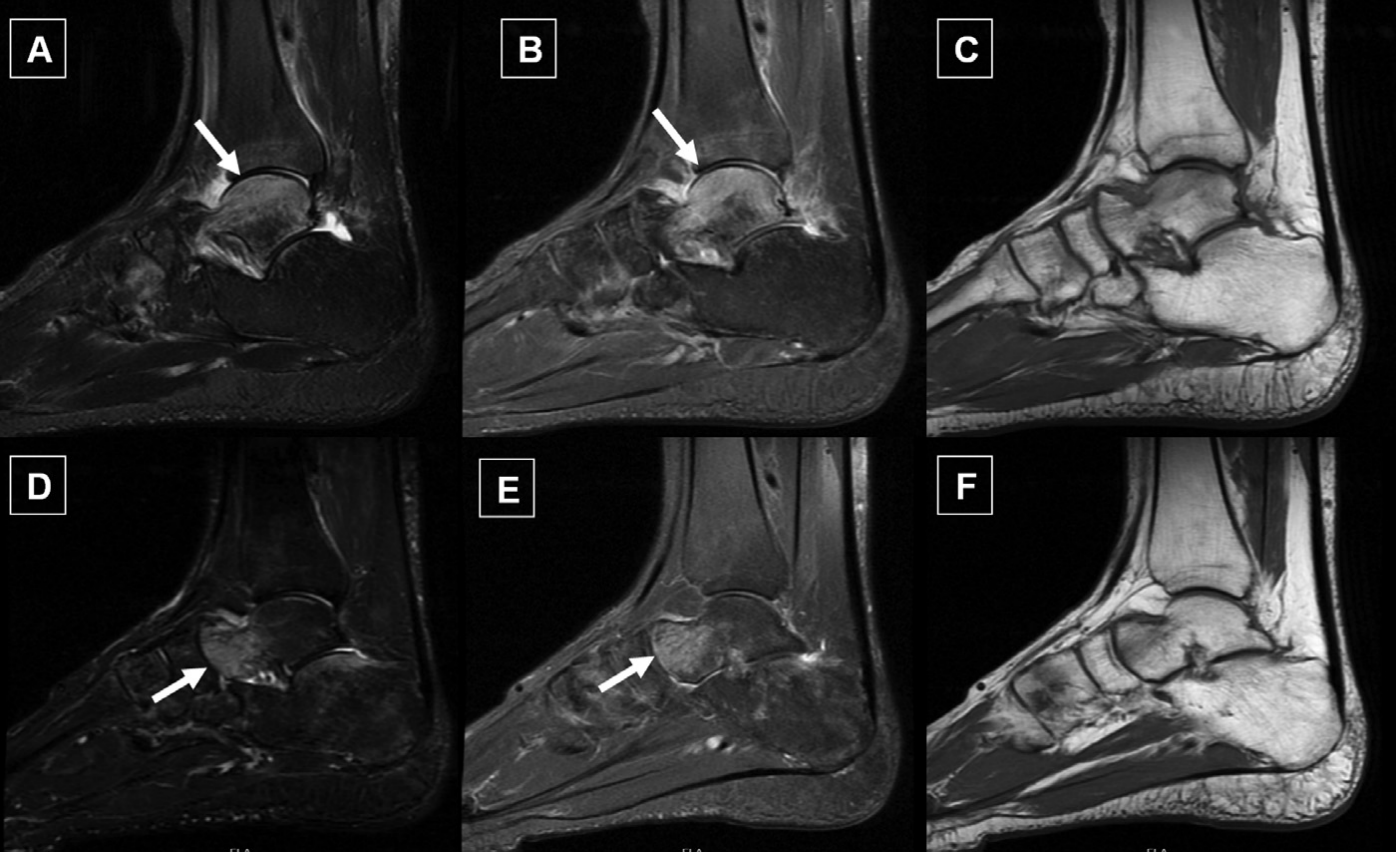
Sagittal MRI planes: (A) STIR, (B) PD, and (C) *T1* sequences of the ankle joint showing intense bone marrow edema (BME) in the dome of the talus bone (white arrows) and dark signal in *T1* (C); Sagittal MRI planes of the same ankle joint, (D) STIR, (E) PD, and (F) *T1* sequences, show that the BME shifted anteriorly in a follow-up MRI, after 6 months of treatment and partial clinical recovery (white arrow).
